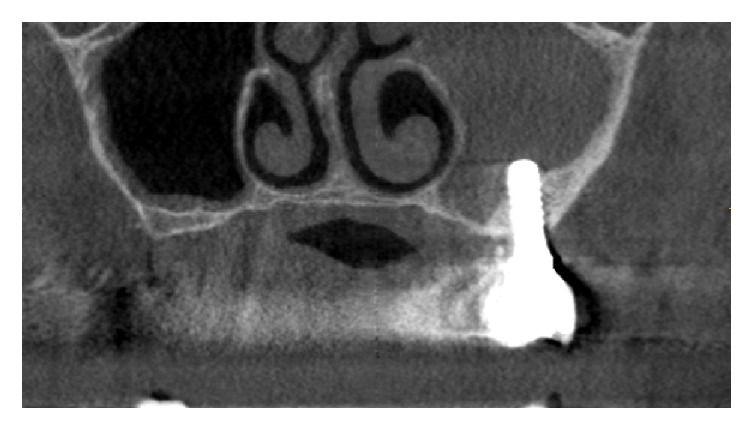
Six months after the removal of the implant 25. The cone-beam computed tomography image revealed that the opacity of the left sinus was still present.
